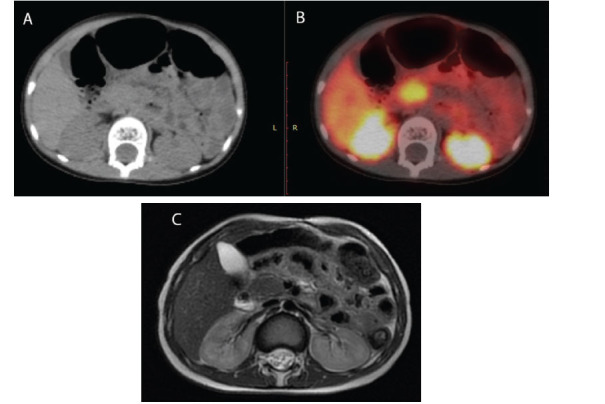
A 3-year-old boy was diagnosed with stage III neuroblastoma of the right adrenal gland (Case 4). The lesion was surgically removed and chemotherapy and radiotherapy were performed according to routine protocol. Hypertrophy of the pancreatic uncinate process and significant uptake on Ga68-DOTATATE PET/CT scan were noted (SUVMax: 7.75). It cannot be distinguished from physiological imaging and an MRI scan was performed. There were no diffusion abnormalities corresponding to the PET/CT scan site and the images were interpreted as normal appearance of the pancreatic tissue. The patient was followed up with no evidence of relapse. (A). CT scan (B). Ga68-DOTATATE fusion image (C). DW-MRI image.
